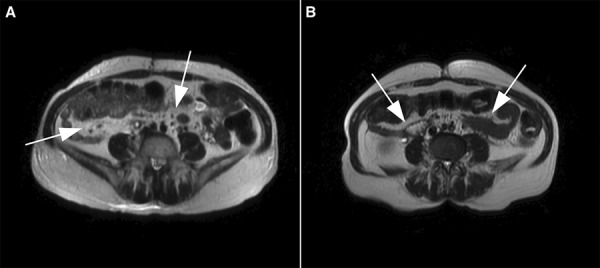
Axial T2-weighted magnetic resonance imaging (MRI) of the pelvis showing the amount of soft tissue before (A) and after (B) therapy. A: There is increased soft tissue in the retroperitoneum inferior to the aortic bifurcation, the cause of the bilateral ureteral obstruction. B: The amount of soft tissue in the retroperitoneum distal to the aortic bifurcation has markedly decreased. There is mild reduction in the right hydronephrosis and significant reduction in the left hydronephrosis. White arrows point to areas of retroperitoneal fibrosis.
